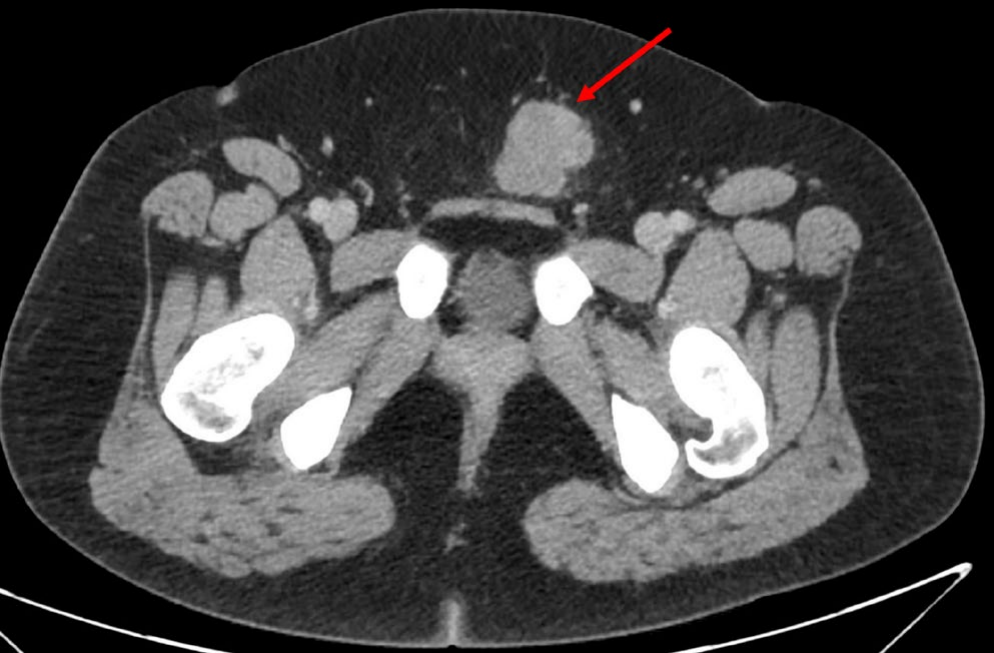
Follow‐up CT abdomen and pelvis with IV contrast performed approximately 1 month prior to the patient's diagnosis. There is interval enlargement of the anterior abdominal wall mass now measuring 3.2 × 2.8 cm with a minimal amount of surrounding fat stranding (red arrow), with the mass showing increased lobulation of the margins. This was thought at the time to represent either a solid mass or hematoma, and ultrasound was recommended to evaluate the vascularity of the lesion.
